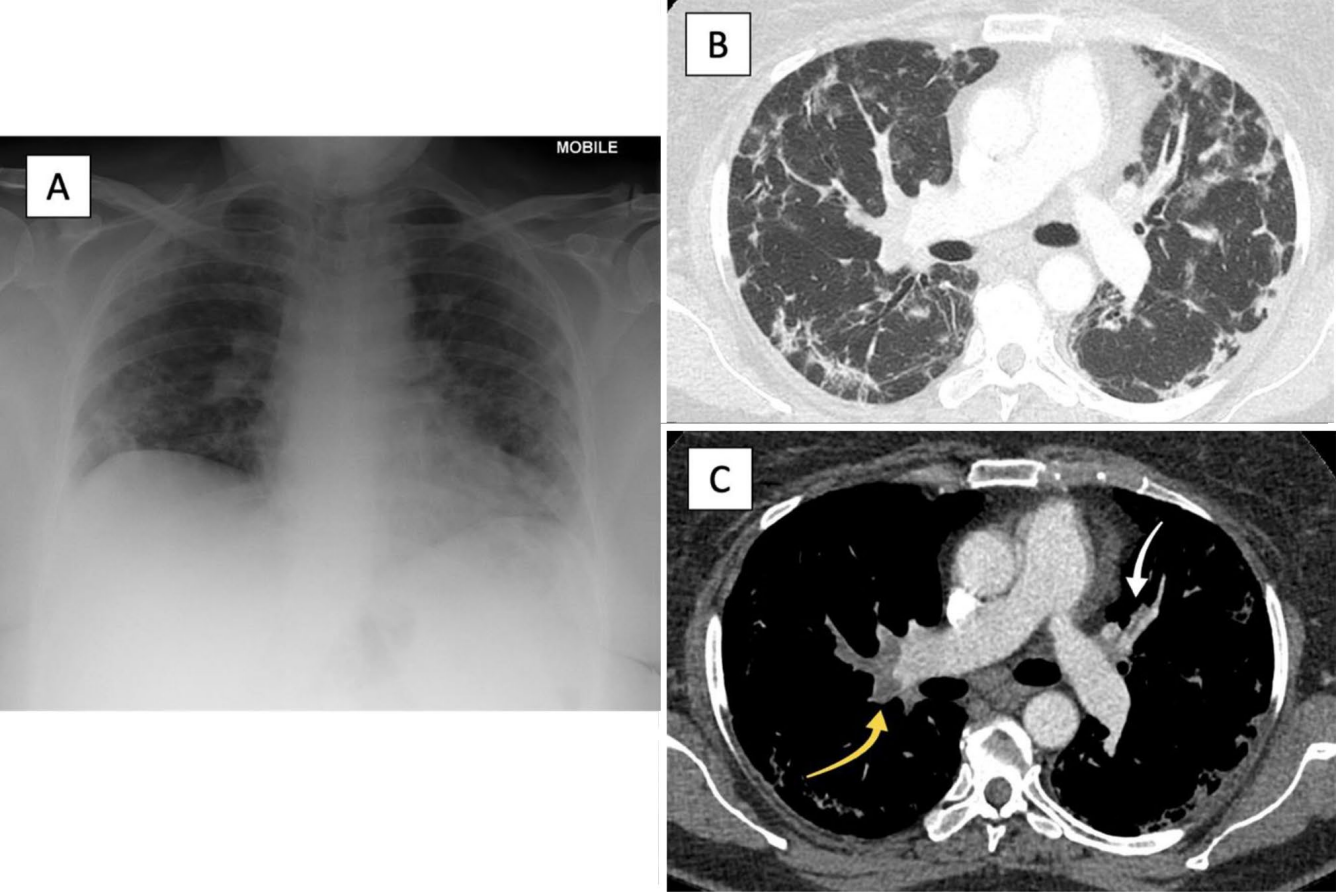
Thoracic imaging of patient with COVID-19 pneumonia with associated pulmonary emboli. Patient managed for COVID-19 pneumonia and pulmonary emboli. Images courtesy of Radiology Department, East and North Hertfordshire NHS Trust. a Chest X-ray showing bilateral peripheral consolidation with mid and lower zone predominance, compatible with COVID-19 pneumonia. b CTPA lung window showing organising pneumonia pattern typical of COVID-19 pneumonia with peripheral consolidation in a perilobular pattern. c CTPA mediastinal window showing large pulmonary embolism within distal right main pulmonary artery (yellow arrow) and small left upper lobe pulmonary embolism (white arrow)
